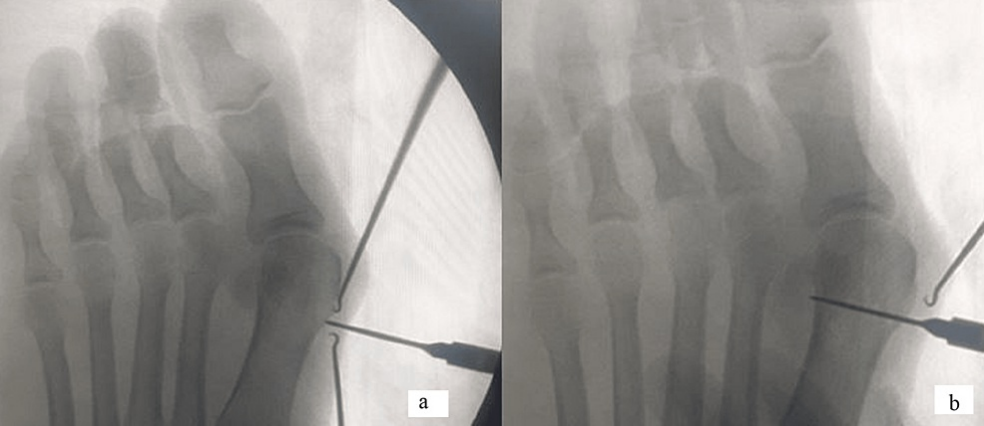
(a and b) Sub-capital osteotomy being performed with an ultrasonic bone scalpel.
